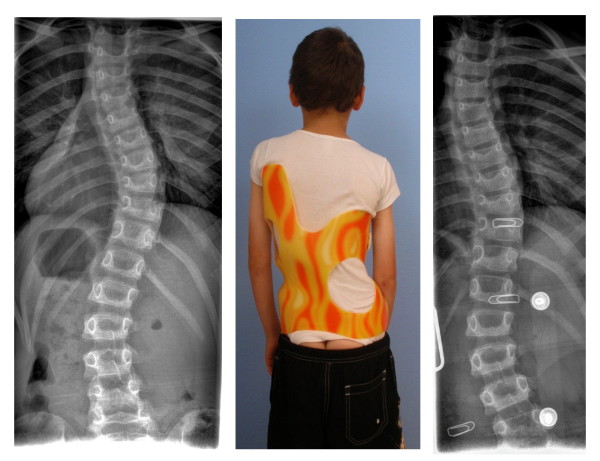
10-year old boy with EOS (early onset scoliosis) in his first Gensingen brace™. Clinically in this boy the flatback is clearly visible. The trunk is rather balanced because of the re-compensation of the 39° thoracic curve by a structural high thoracic counter curve. The curve is very stiff and has a bad prognosis, which can be estimated from the x-ray: In the apical area of the thoracic curve a significant wedging of the vertebra is visible. Nevertheless the curve has been corrected to 18° in the brace, which is sufficient considering the fact that double thoracic and EOS curves cannot be corrected as easy as single thoracic curves in patients with AIS. The foam pad location is marked on the in-brace x-ray with wire as is the longitudinal width of the thoracic pad.
